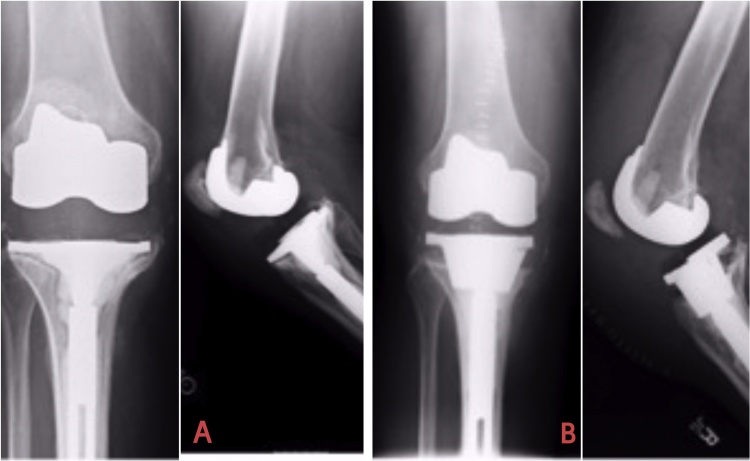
Fig. 1A=Preoperative Xrays of Journey II twice previously operated knee with aseptic osteolysis and loosening of the Revision Tibia component which was revised to Legion revision tibia with proud Tibial cone without augments. Fig. 1B depicts radiographs at 5 years follow-up.
